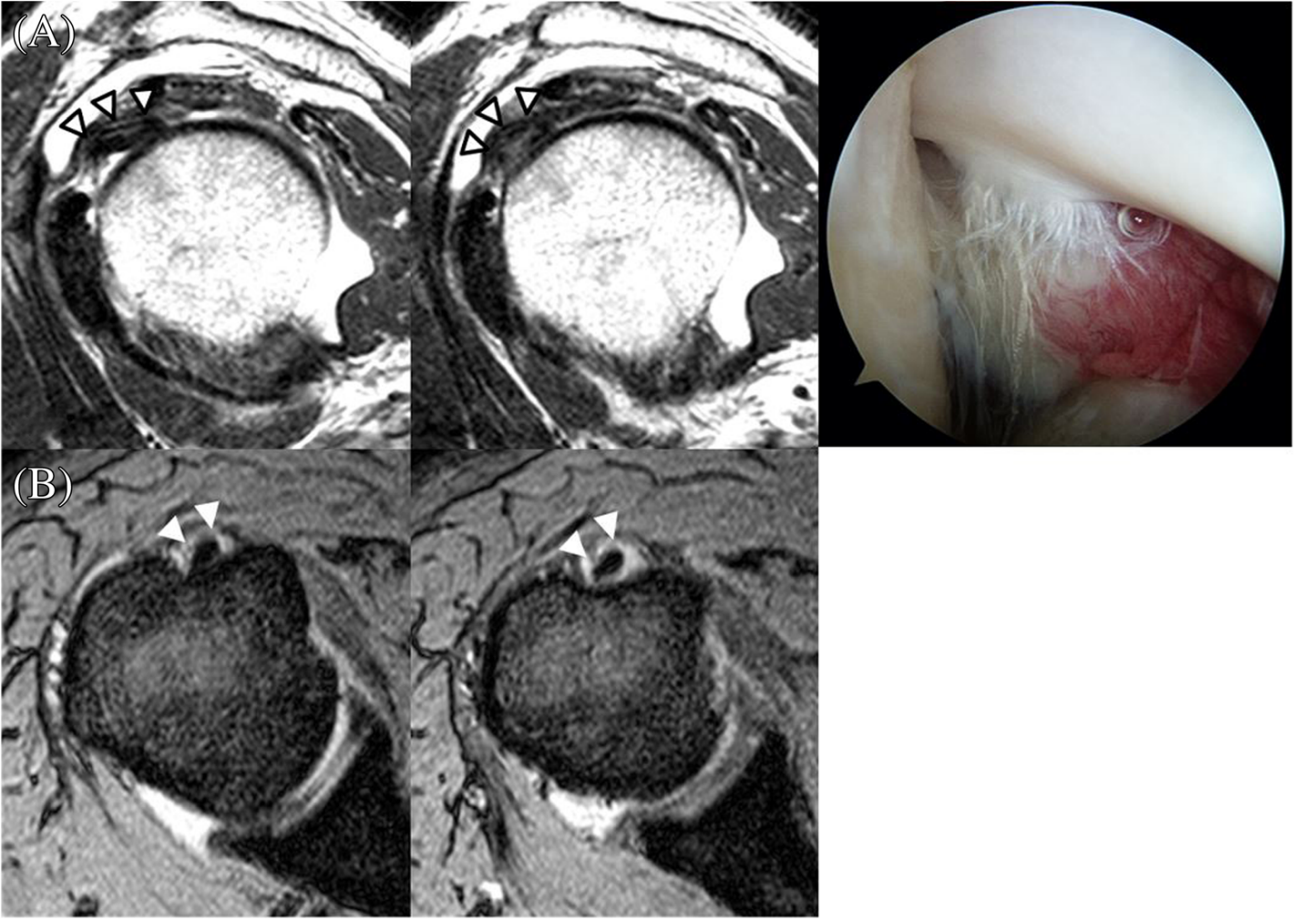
a Two consecutive T2-weighted parasagittal MRI showing mild contour irregularity and heterogenous high signal intensity inside the tendon (white arrowhead). b Two consecutive T2-weighted axial MRI of the same patient showed high signal intensity surrounding the tendon, suggesting effusion in bicipital groove (Type III)
